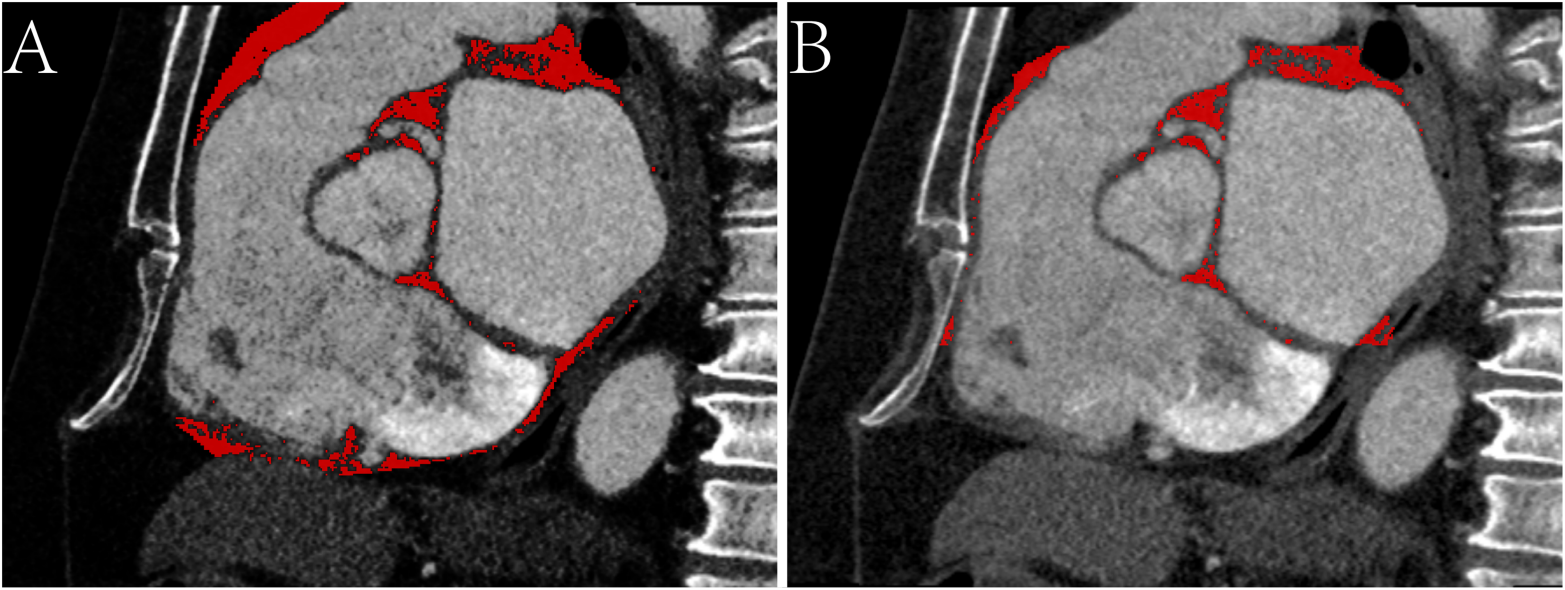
Sagittal CT images of the epicardial adipose tissue on -200 to 0 HU. (A) The red area represents the epicardial adipose tissue (EAT) around the whole heart. (B) The red area represents the EAT around the atrium.
